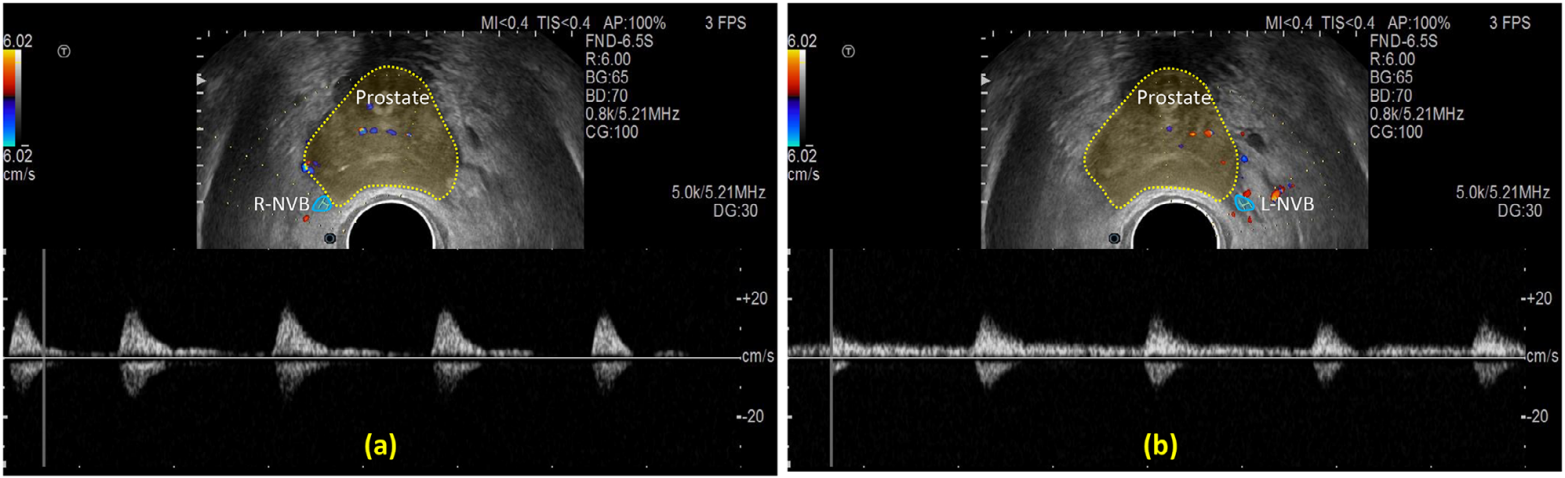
An example of a patient's pulsed wave Doppler measurements of (a) right and (b) left NVBs. The prostate is outlined with a dotted yellow line, and the right and left NVBs are outlined with blue lines in the ultrasound images. The pulsed wave Doppler signals are below the ultrasound images.
